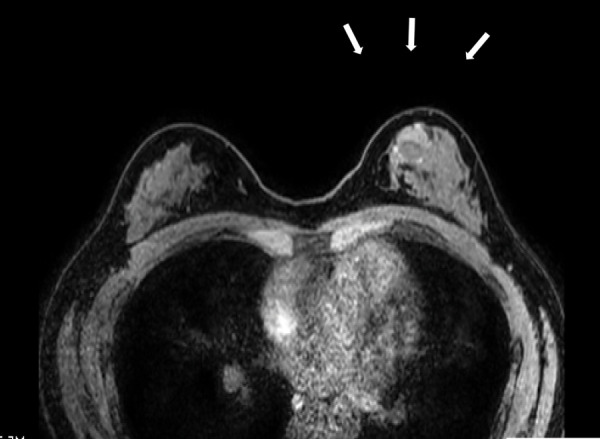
MRI. MRI examination showed a space-occupying lesion measuring approximately 2 cm in diameter in the upper-inner quadrant of the left breast as indicated by the arrows. There were no findings suggestive of obvious lymph node enlargement. The lesion was well defined, and a cystic mass was seen.
